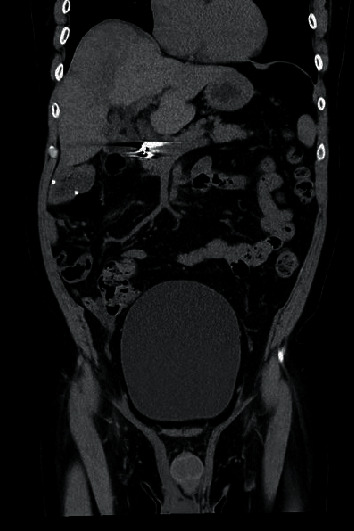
Noncontrast CT abdomen in coronal plane demonstrates enlarged bladder to the level of the umbilicus.
